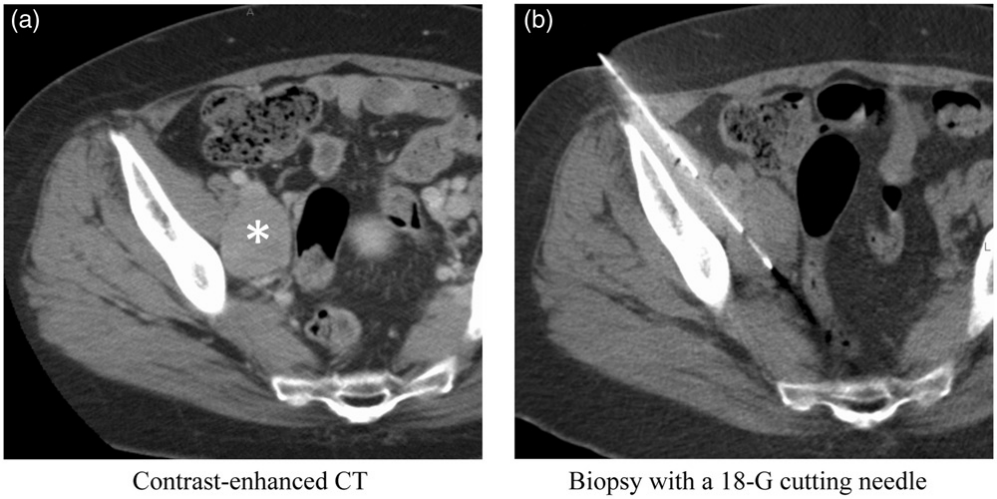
Representative case showing biopsy of a pelvic mass from a woman in her 60s. (a) Contrast-enhanced CT of the pelvis demonstrates an enhanced mass (asterisk) between the right external and internal iliac vessels. (b) Biopsy was performed using an 18-G cutting needle without penetration of the iliac arteries and veins.
